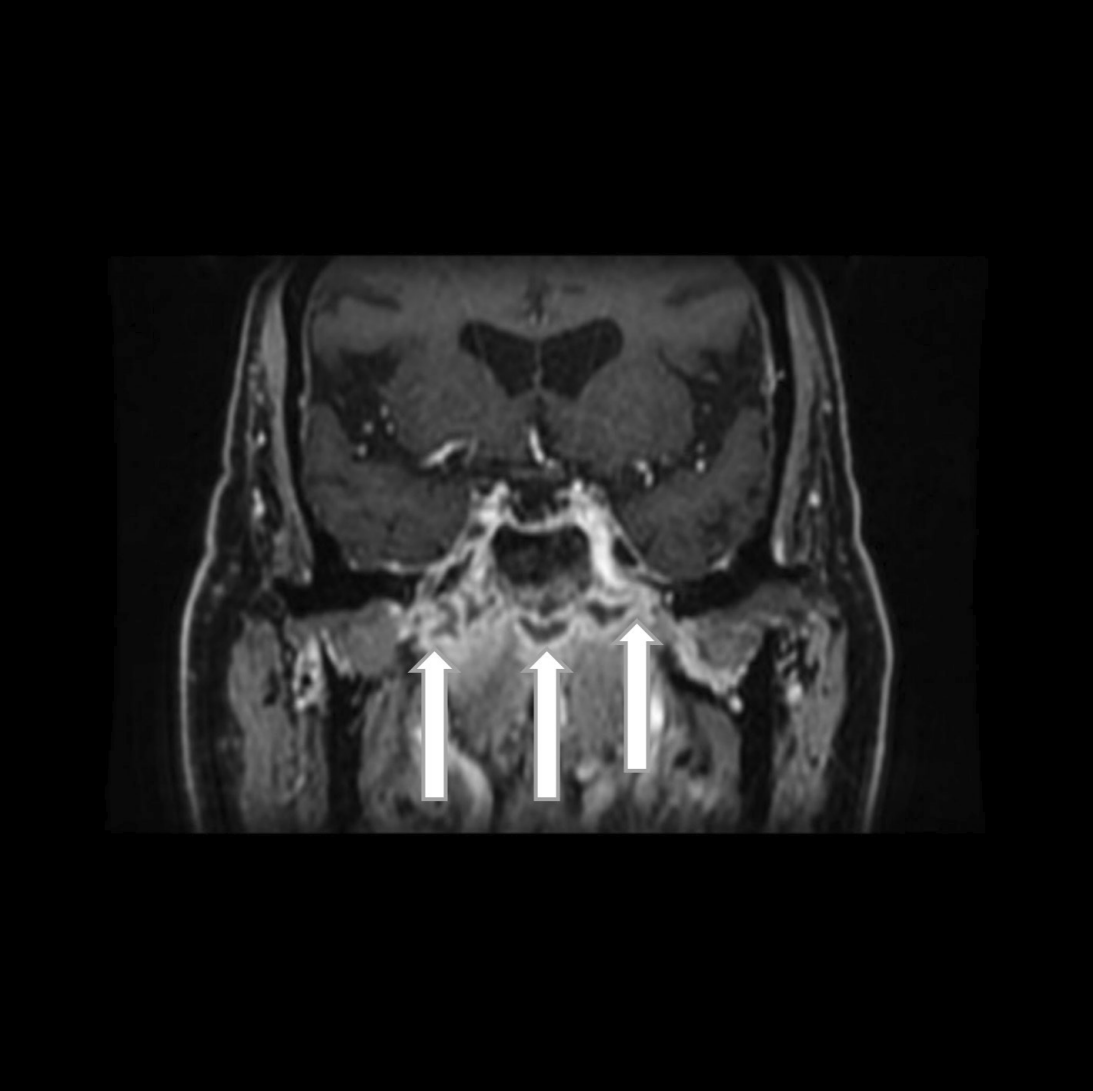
Coronal T1‐weighted MRI scan showing bilateral contrast‐enhancement along the middle skull base with some degree of necrosis (simple arrows).
